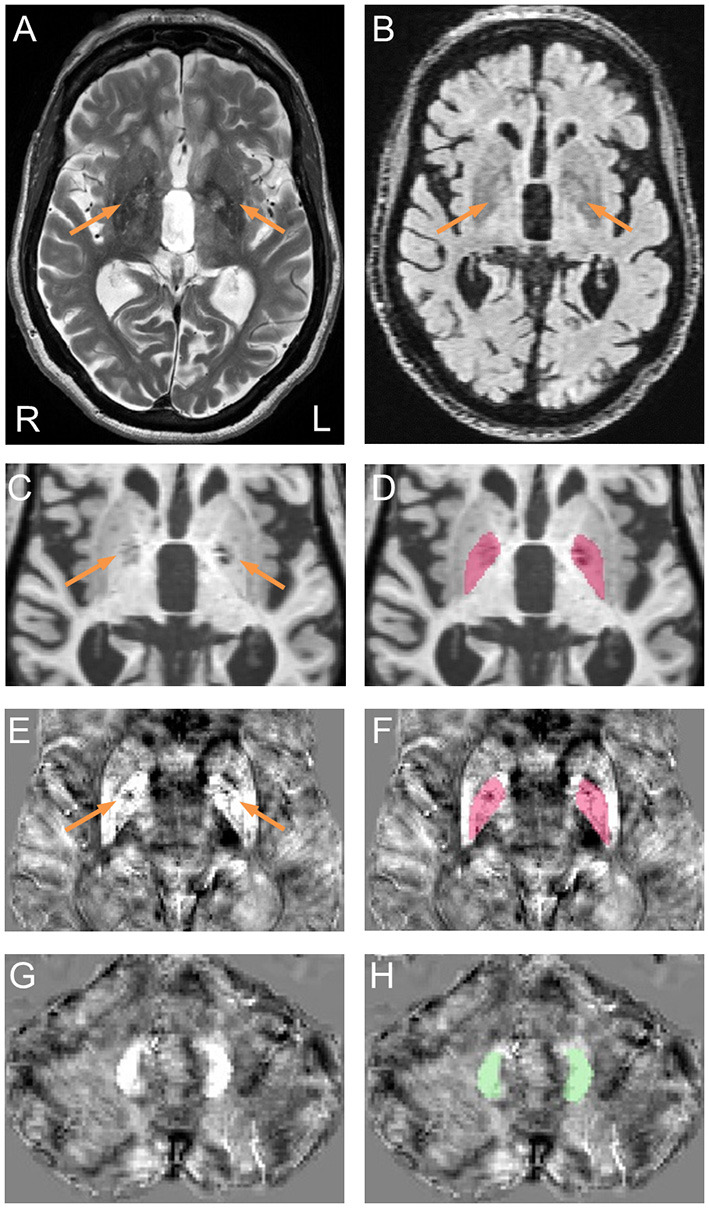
Representative pallidal T2-abnormalities and segmentation of the globus pallidus and dentate nucleus. (A) Pallidal T2-abnormalities (arrows) on a T2 scan acquired from a male premutation carrier who visited 5 times from age 62 to 74 years. The patient had action tremor and cerebellar ataxia that started at age 56 and “*Definite*” FXTAS diagnosis of at all visits. Behavioral Dyscontrol Scale scores decreased from 17 (visit 1) to 12 (visit 2) and to 10 (visits 3 and 4) and Symptom Checklist-90-Revised Global Severity Index scores increased from 56 (visit 1) to above 70 at visits 3, 4, and 5. (B) FLAIR scan shows hyperintensive signals in the central pallidal region (arrows) that correspond to the region showing hyperintensive signals in the T2 scan. (C) T1 scan shows bilateral signal loss in the globus pallidus (arrows). (E,G) QSM image co-registered to T1 scan, shows variation in susceptibility in the globus pallidus [(E), arrows] but not in the dentate nucleus (G). (D,F) Segmentation of the globus pallidus on T1 (D) and QSM (F). (H) Segmentation of the dentate nucleus on QSM.
